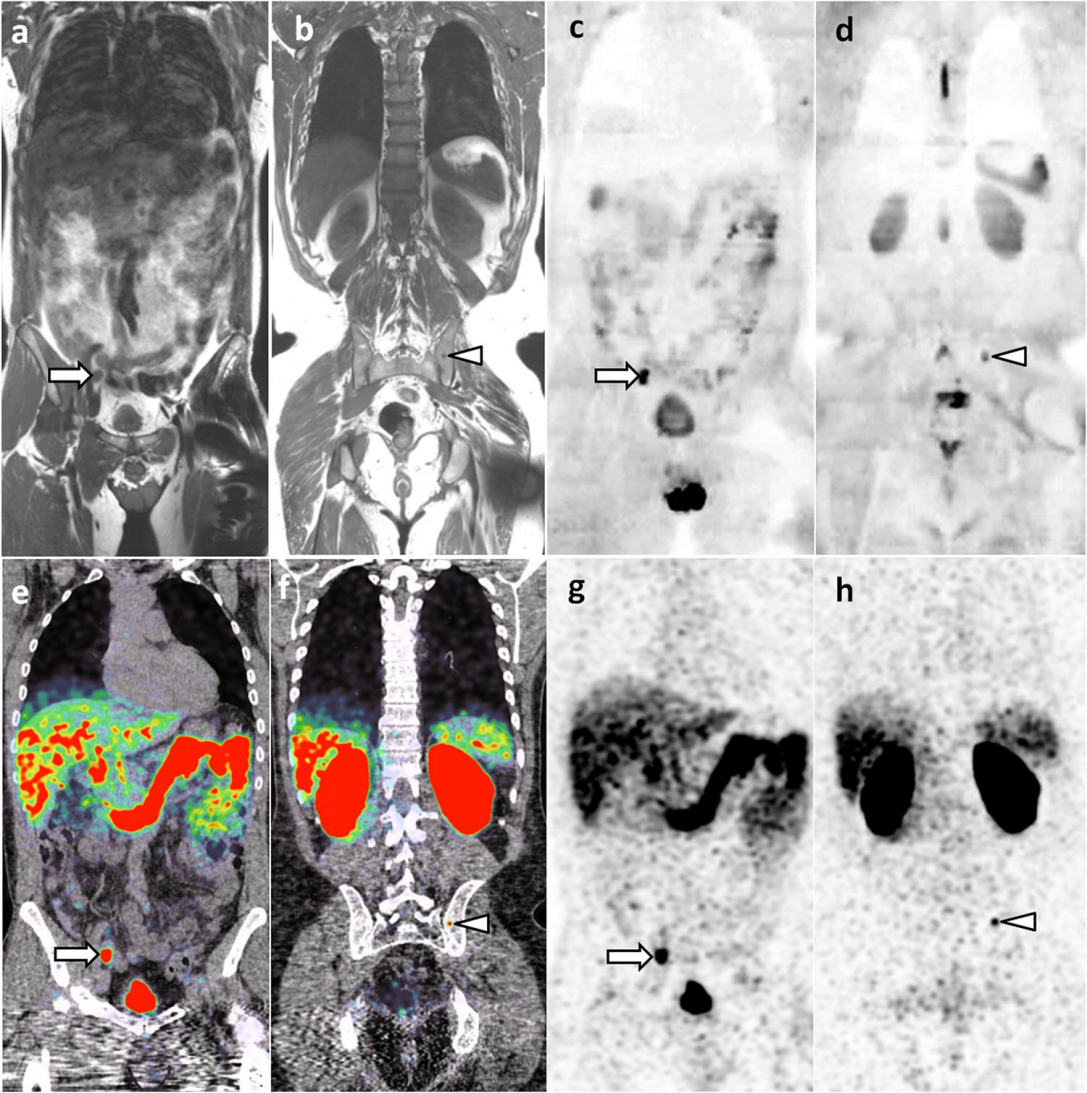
69-year-old man with newly diagnosed prostate cancer: comparison of WB-MRI and PSMA-PET/CT findings. a–d Coronal WB-MRI T1- (a, b) and high *b*-value (inverted grayscale window, *b* = 1000 s/mm^2^) DWI-weighted (c, d) images show right iliac lymph node metastasis (arrow) and left iliac bone metastasis (arrowhead). e–h Corresponding coronal images of PSMA-PET/CT (PET/CT fusion (e, f) and PET (g, h)) show the same lymph node (arrow) and bone (arrowhead) metastases
